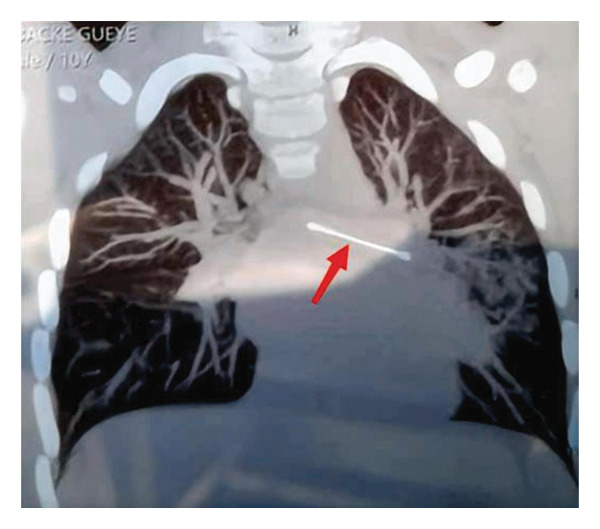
Chest computed tomography showing a striated hyperdense shadow in the left main bronchus (red arrow).
